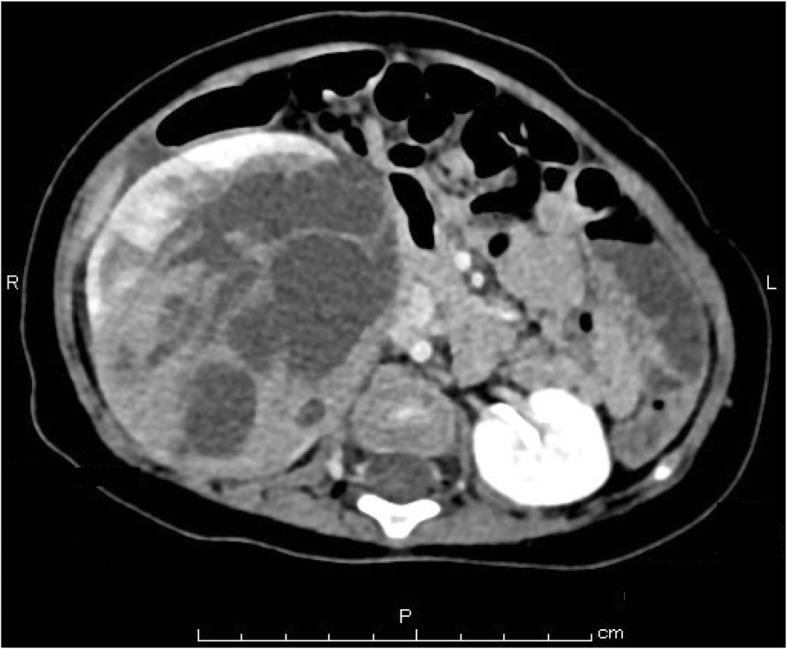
Enhanced abdominal CT. Multilocular cystic mass located in the right kidney. The septal wall of the tumor was thickened. Multiple solid areas were identified within the tumor
